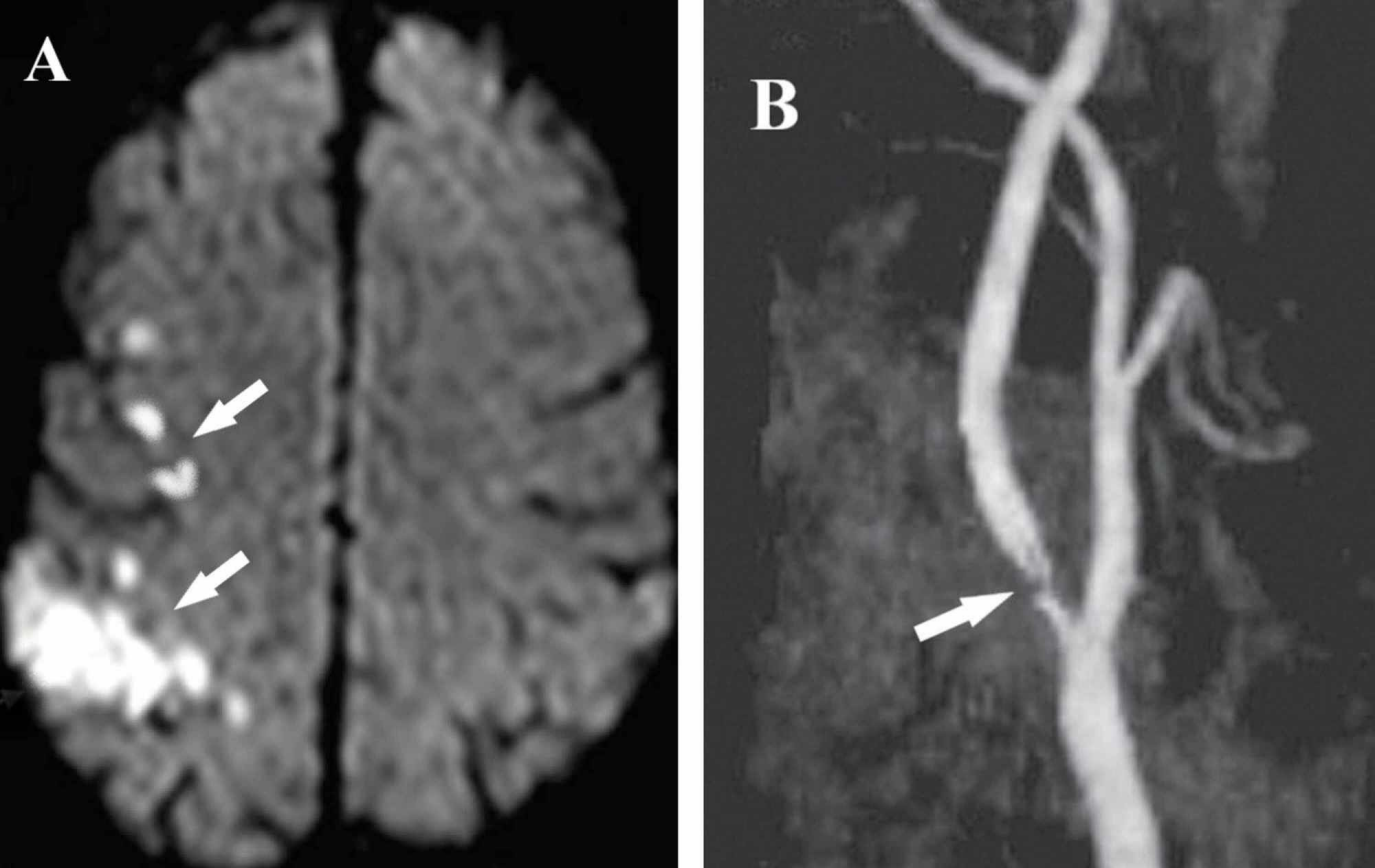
(A) Axial MRI diffusion sequence reveals multiple watershed ischemic lesions (white arrows) involving the frontoparietal cortex. (B) Magnetic resonance angiography of right internal carotid artery showing severe proximal stenosis at its origin (white arrow).
